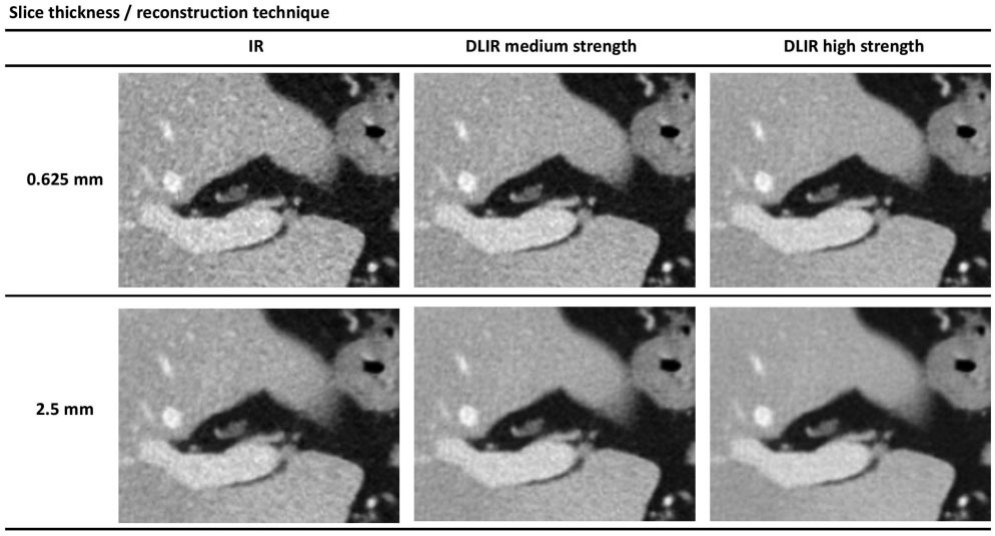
A selection of axial CT of the abdomen images centered on the liver hilum, reconstructed with standardly applied IR, DLIR of medium strength and DLIR of high strength in 0.625 mm and 2.5 mm slice thickness.
